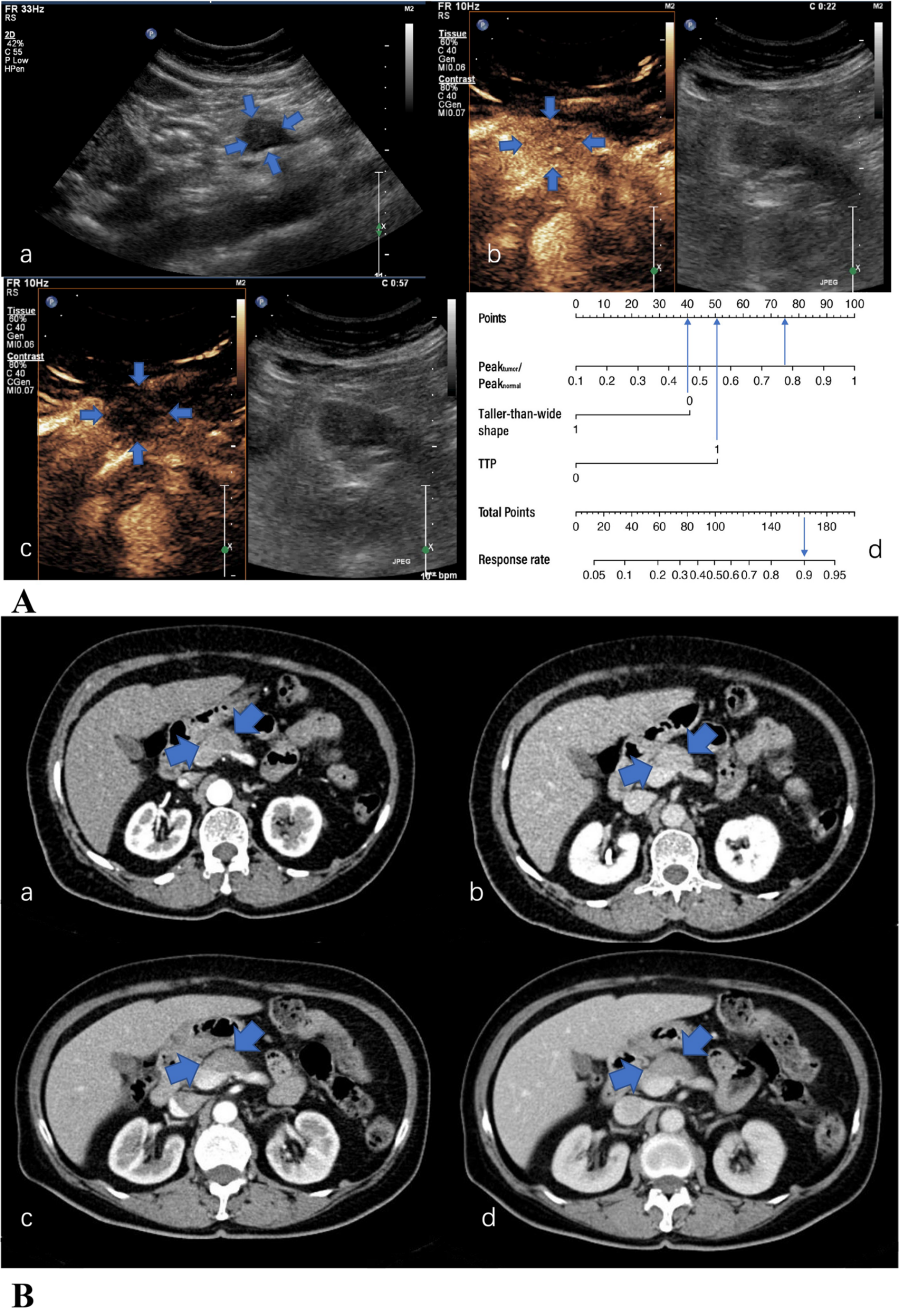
A US and CEUS of a 67-year-old woman with a 3.0 cm hypoechoic solid lesion in the head of the pancreas. a The lesion was not in a taller-than-wide shape in the longitudinal plane. b The TTP was 24 s and the Peak_tumor_/Peak_normal_ was 0.772 (in the transverse plane). c The lesion in the venous phase (in the transverse plane). d A total of 170 points were assigned to the lesion according to the nomogram, corresponding to an about 90% probability of “responders”. After 4 cycles of NAC, a radiographic response of SD was achieved. B The pre-treatment (a, b) and post-treatment (c, d) CT images of the case. CA 19–9 decreased from 50.8 to 20.3 U/mL
